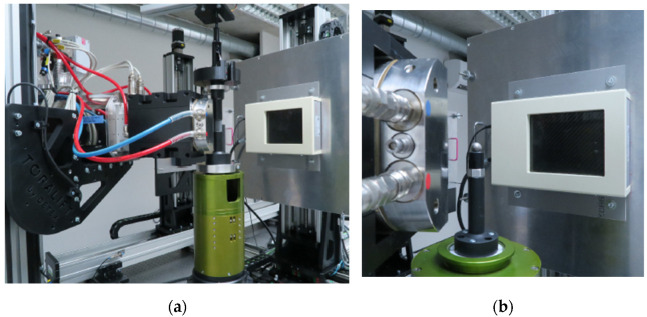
Experimental setup comprising the in-situ loading device mounted in the CT scanner: (a) An overview showing the X-ray source, the loading device on the rotary stage, and the detector; and (b) detail of the loading device with the demounted carbon-fibre loading chamber with an emplaced APM sample.
